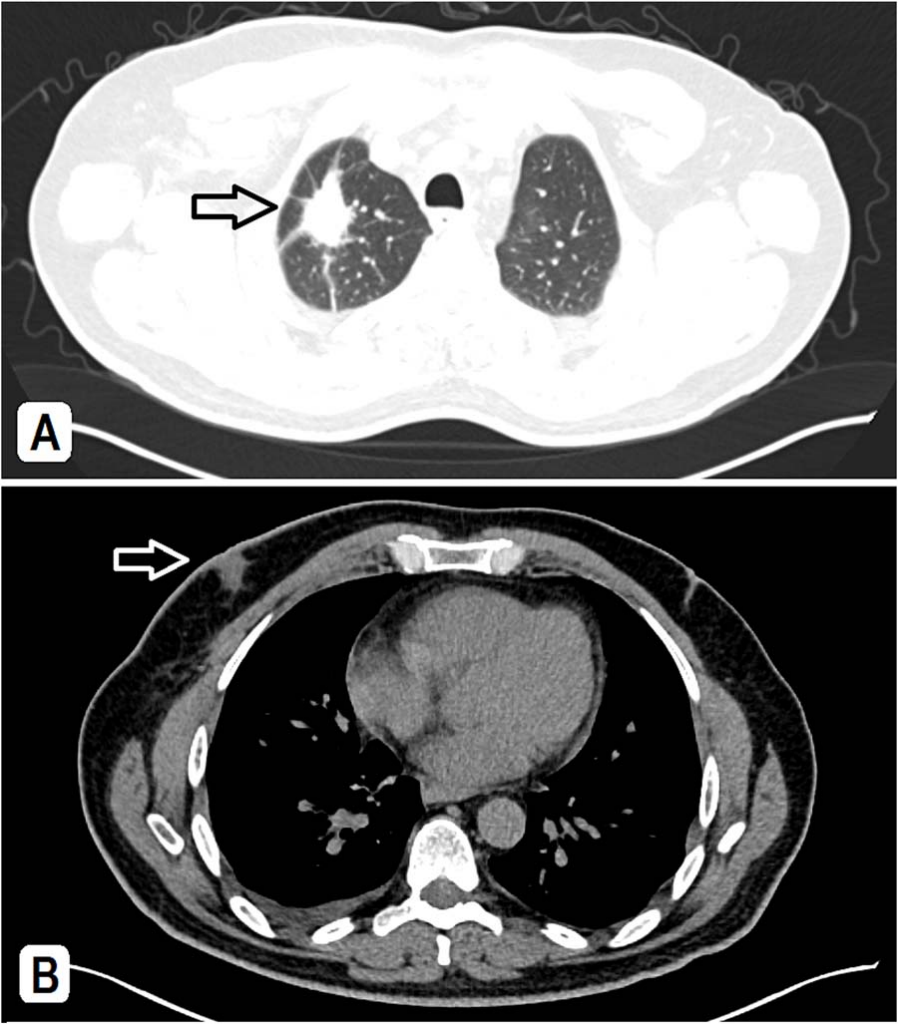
(A) A CT scan showed a spiculated lesion in the right lung apex with adjacent atelectatic bands, pathologically enlarged hilar, mediastinal, and right-sided axillary lymph nodes, the largest measuring 1.5 cm. (B) The mediastinal window of CT at the chest level shows an asymmetry of breast tissue with right-sided retro-areolar soft tissue density.
